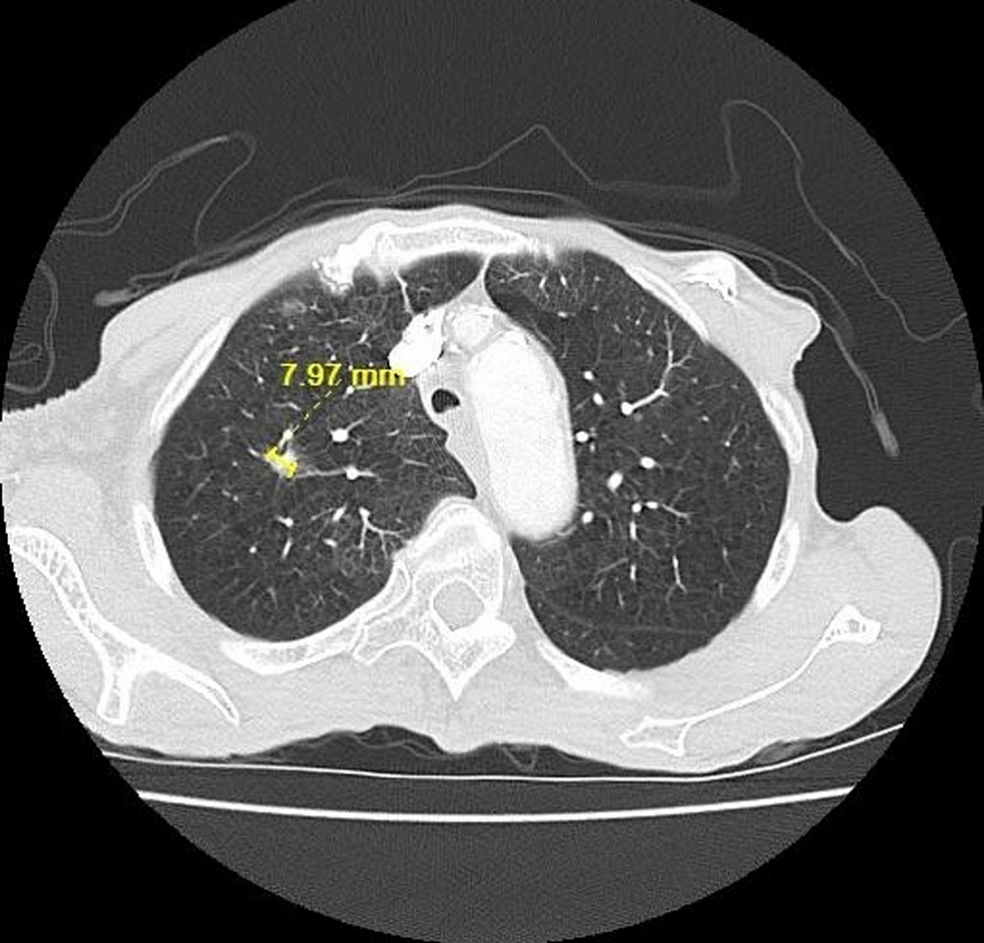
CT chest lung window showing right upper lobe lung nodule during chemotherapy on 11/27/2017
